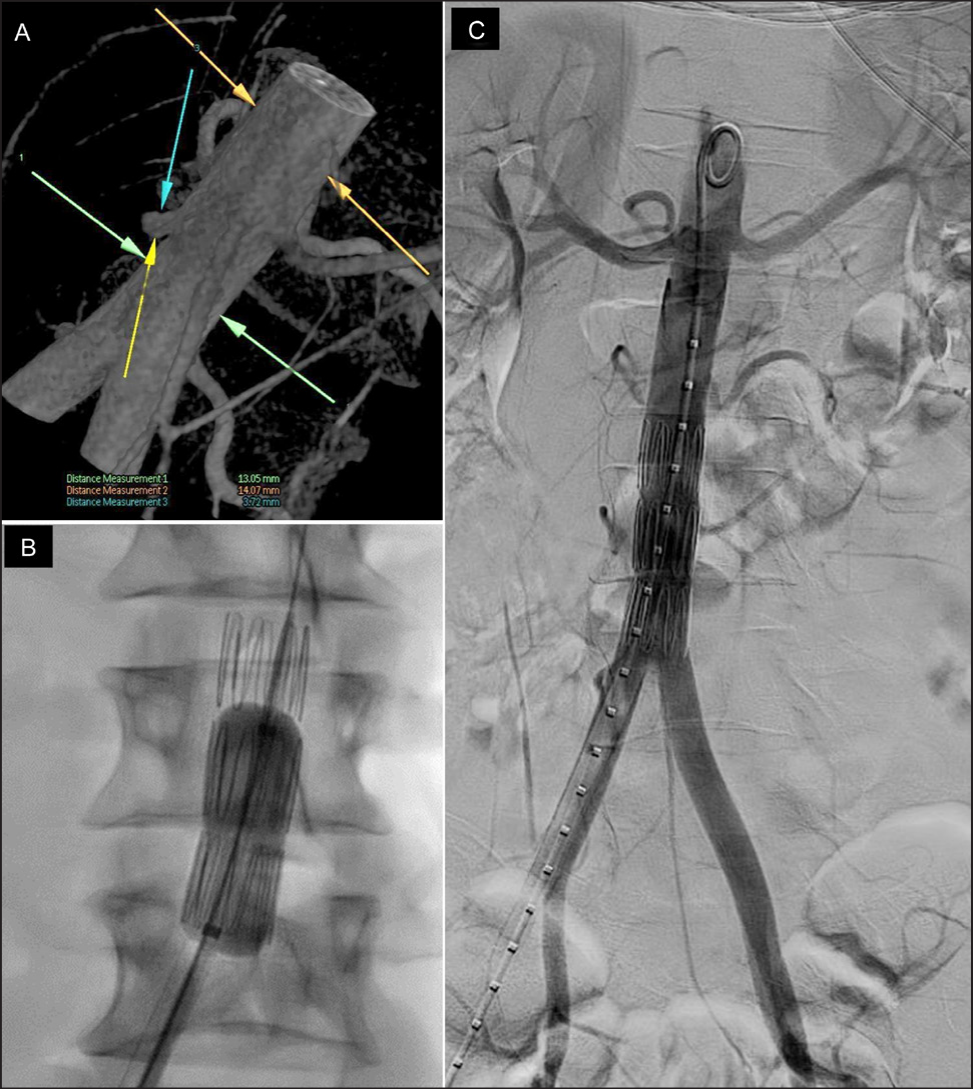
A: 3D(VR) c-arm cone-beam CT view of the aorta, for calibrated measurements, 3D-roadmap and planning of the stenting. The proximal avulsion of the RPIA looks like a 3.7 mm pseudo-aneurysm of the aorta. B: 18 × 56 mm self-expandable stent with 14-mm balloon remodeling. C: Final angiogram showing perfect positioning of the stent, covering both RPIA and LPIA, associated with non-enhancement of the lower pole of both left and right kidney.
